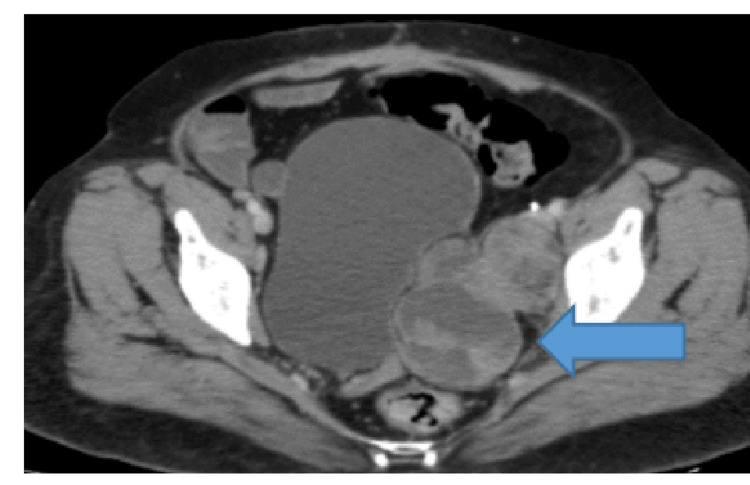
CT scan axial view showing interval increase in size and number of the pelvis side wall masses.
